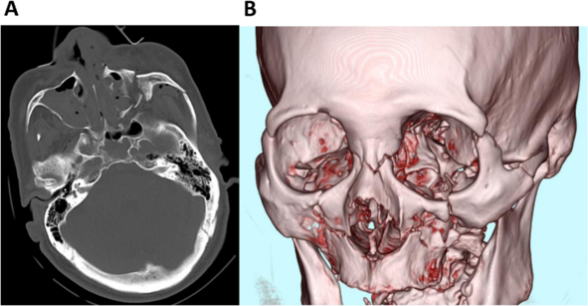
Craniofacial computed tomography scan obtained on arrival. a Bilateral fractures of the maxillary sinus wall and fluid collection were detected. Complex fractures were observed on the patient’s left side. b A three-dimensional computed tomography scan showed fractures extending through the nose, along the floor and medial and lateral walls of the orbit, and across the left zygomatic bone
